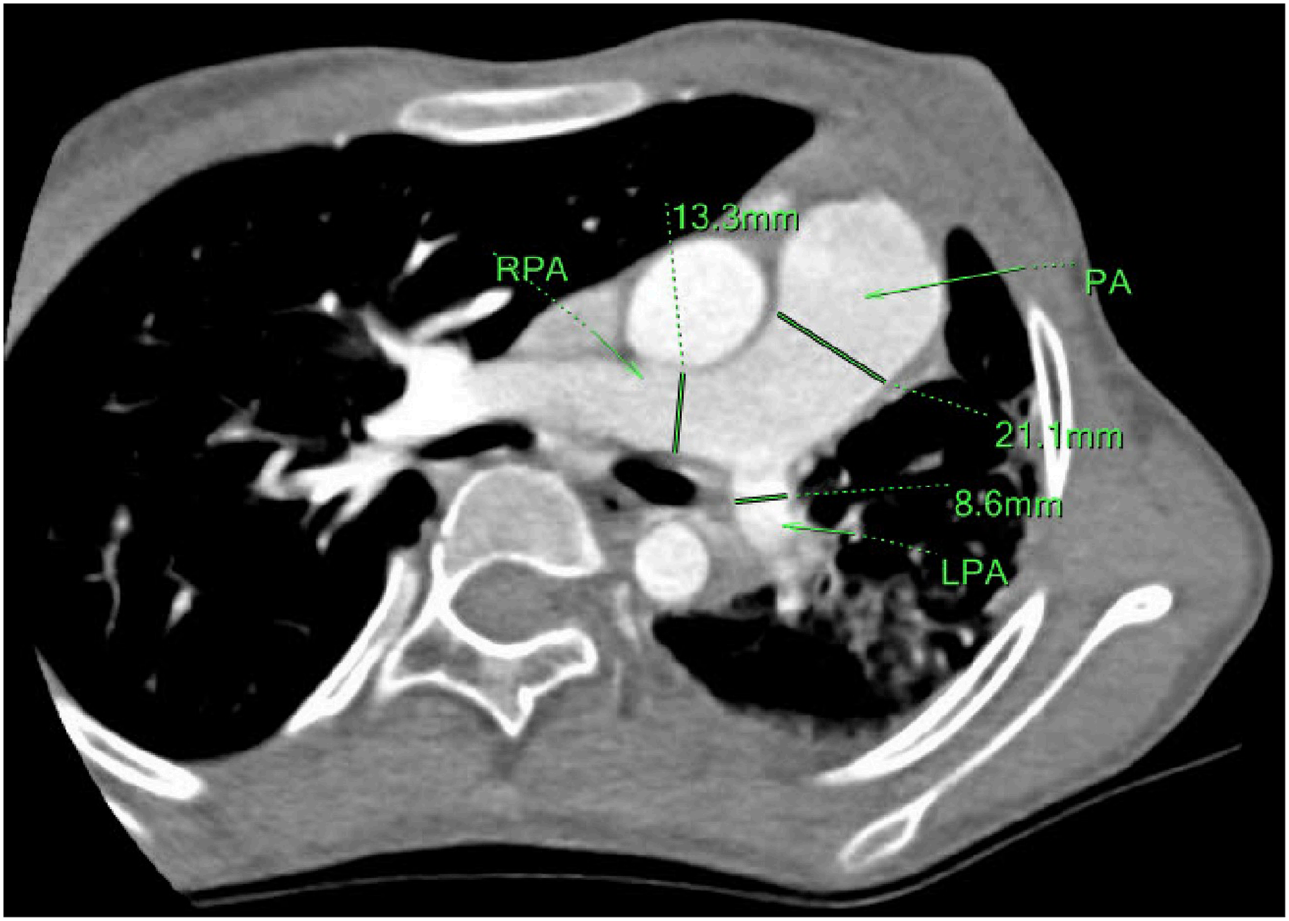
Cardiac CTA (mediastinum window) of an 11-year-old girl at her first admission (Case No. 82), showing hypoplasia of the left lung, thinning of the left pulmonary artery, and dilation of the main and right pulmonary arteries. RPA = right pulmonary artery; PA = pulmonary artery; LPA = left pulmonary artery; CTA = computed tomography angiography.
